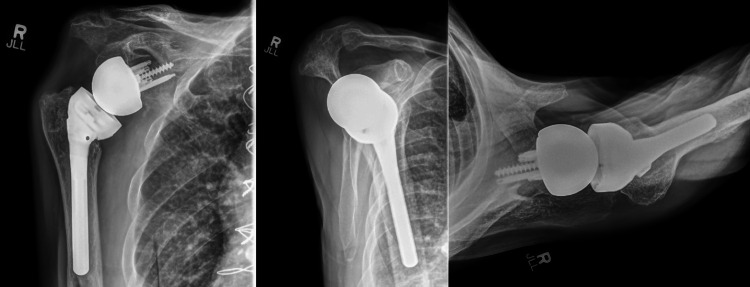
Radiographs of the right shoulder at the four-week postoperative visit demonstrating well-aligned revision reverse shoulder arthroplasty implants
